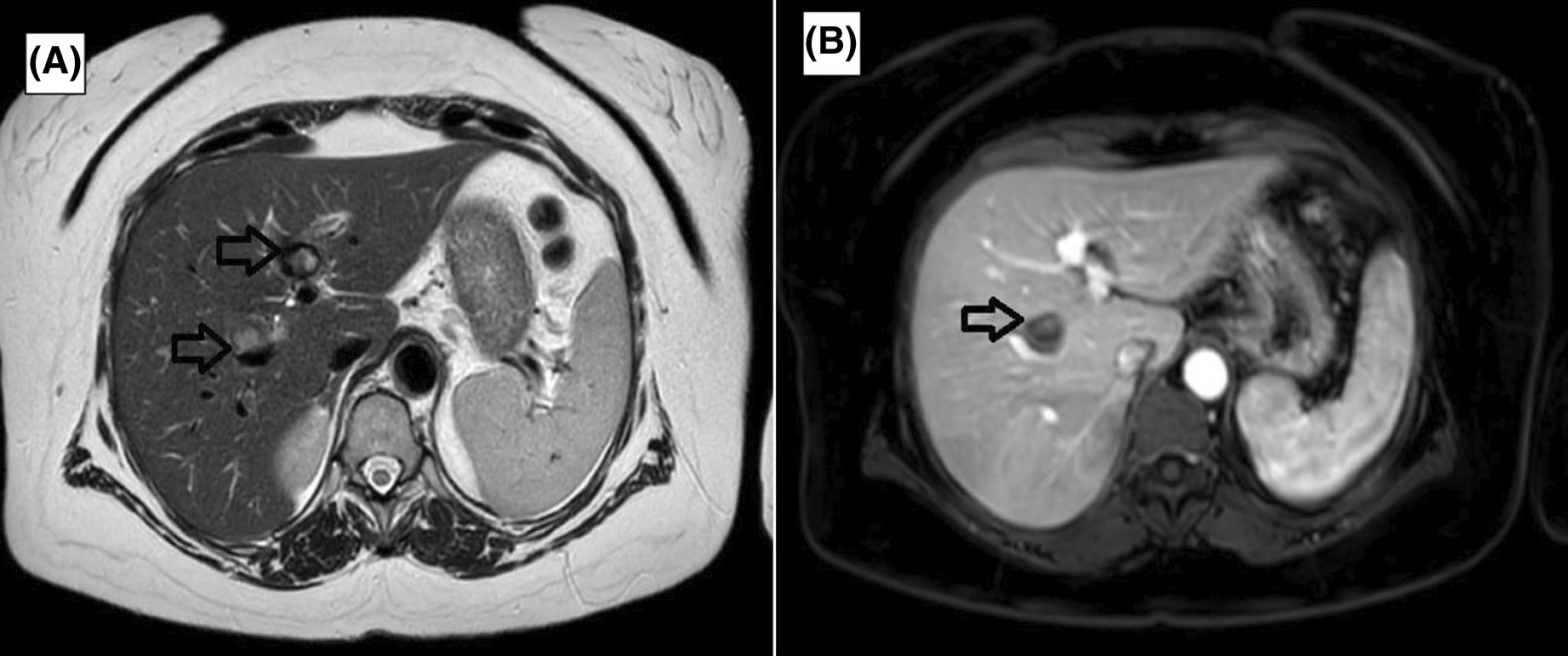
The black arrows show the thrombosed aneurysms in the right and left hepatic arteries on the axial T2W (A) and the contrast‐enhanced T1W magnetic resonance images (B).
